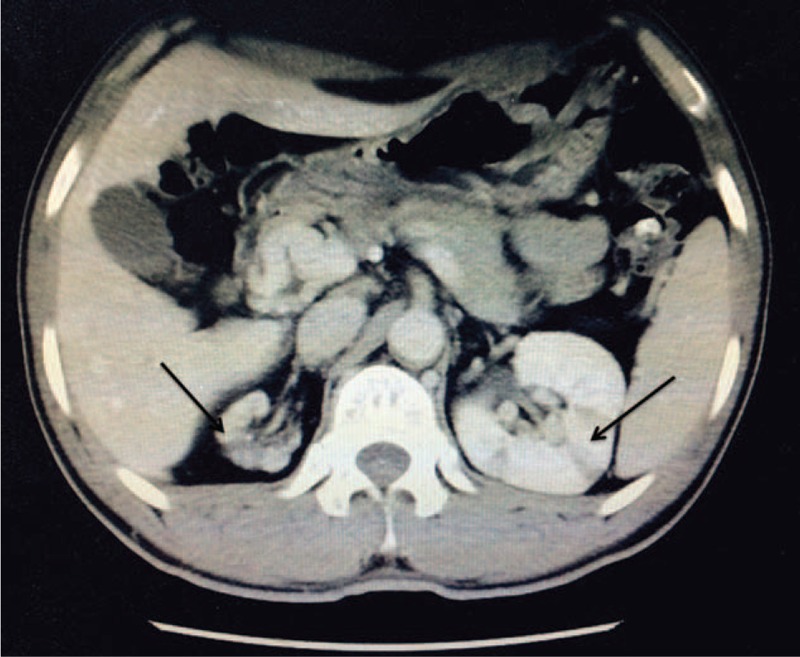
Computed tomography scan showing multiple areas with low-signal attenuation in the right and left renal parenchyma with right kidney shrinkage.
